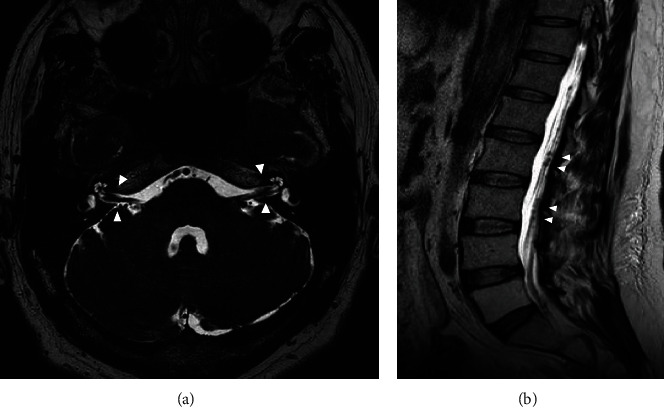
T2-weighted MRI showing vestibulocochlear nerves metastasis (a) and cauda equina metastasis (b) (arrows).
